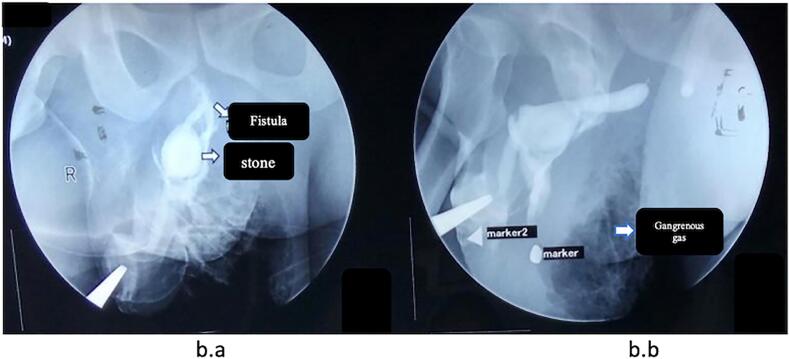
a. Kidney ureter bladder X-ray. b.a. Urethrography in the frontal position. b.b Urethrography in the lateral position.
